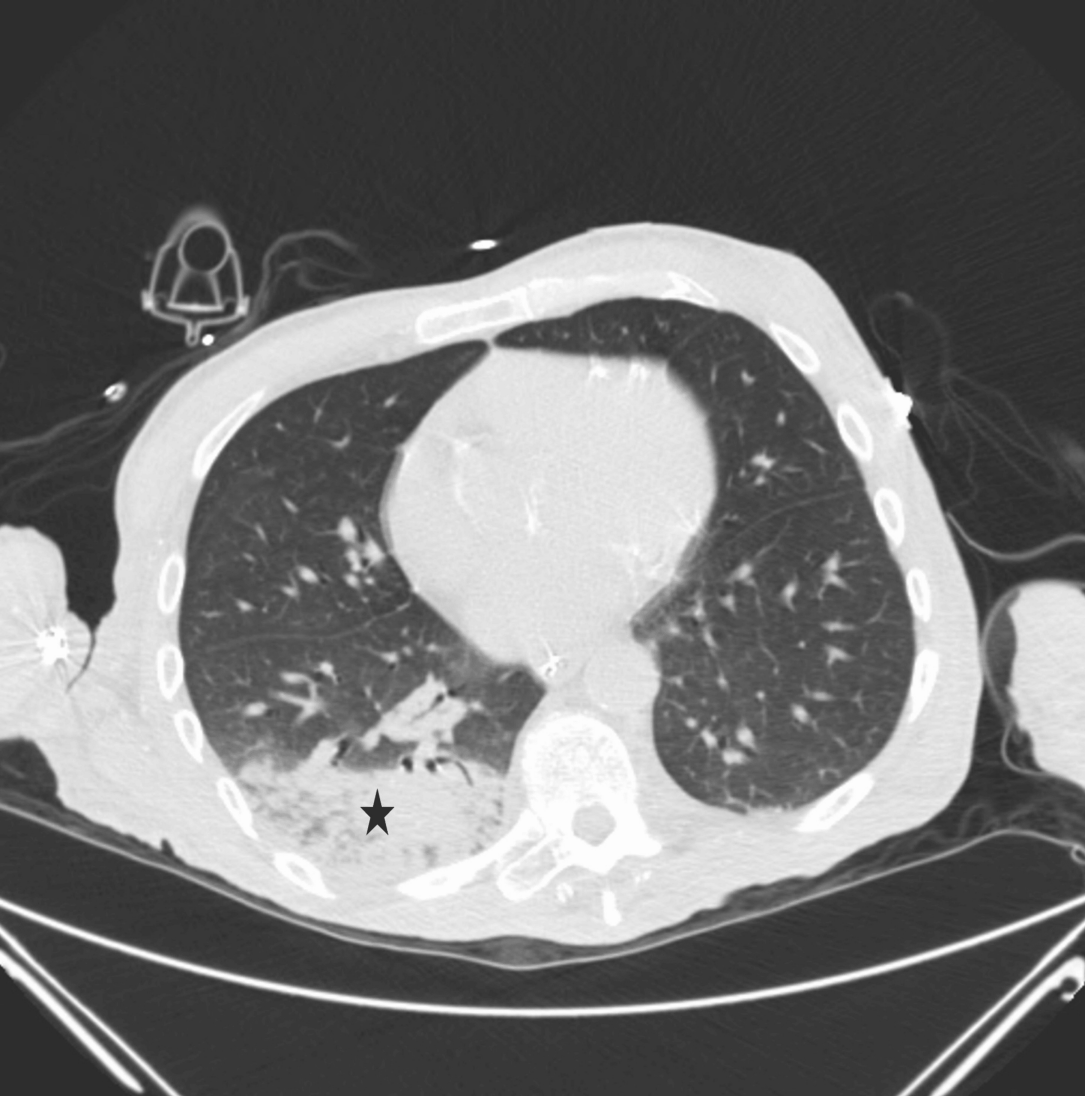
Non-contrast CT of the chest showing large dense consolidation (black star) of the right lower lobe.
